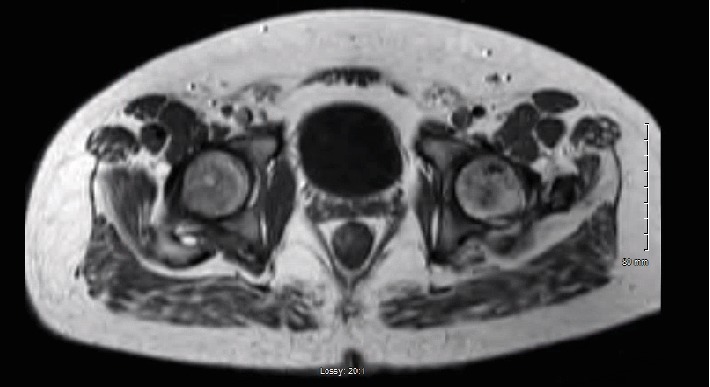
T1 axial image from MRI obtained 7 months postinjury demonstrating focal areas of hypointensity within the left femoral head consistent with osteonecrosis.
